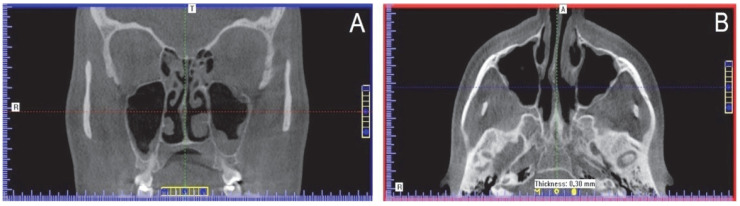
Image of maxillary sinuses after 30 sessions of electromagnetic field and LED light therapy. (A) coronal view; (B) axial view. (CBCT scans).
